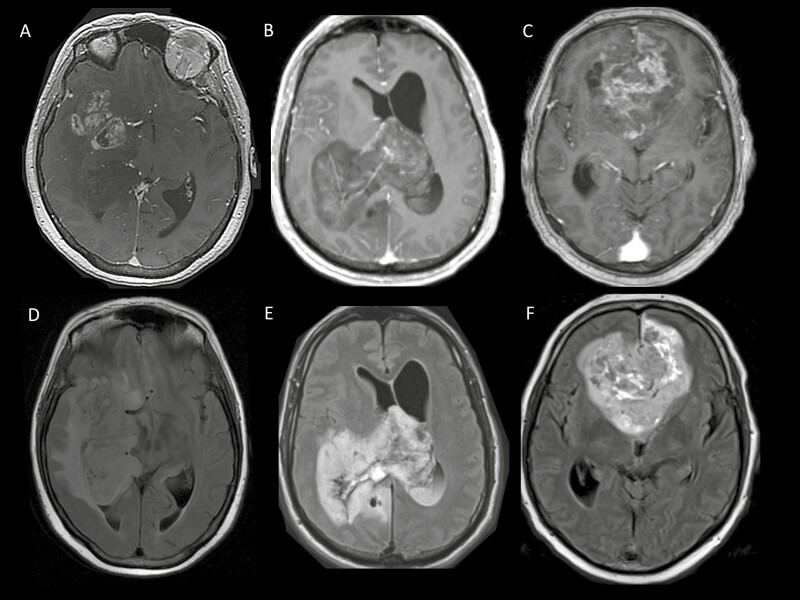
Representative examples of magnetic resonance imaging (MRI) presentation in 3 STS patients. Top: post-gadolinium axial T1-weighted images in 3 different STS patients (A, B, C) Bottom: corresponding axial Fluid-attenuated inversion recovery (FLAIR) (D, E, F).
